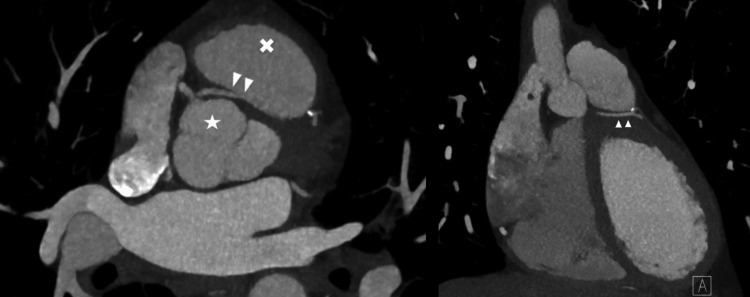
Coronary computed tomography angiogram findings of single coronary artery Coronary computed tomography angiogram showing single coronary artery arising from right coronary cusp (star). The anomalous left coronary artery (arrowhead) travels between the right ventricular outflow tract (x) and the aorta.
